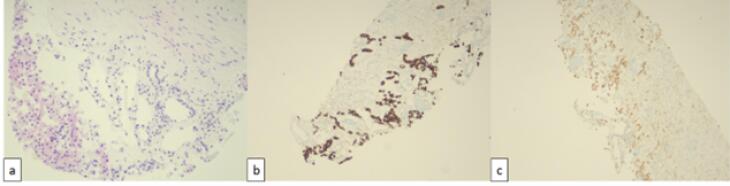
Based on these findings, we diagnosed stage IV disease ChRCC. The patient was referred for ophthalmology consultation because of ocular symptoms. A fundoscopic examination revealed limited lateral gaze in the right eye as a result of invasion of the rectus lateralis muscle in the retro-orbital region. Sunitinib was administered orally at a dose of 50 mg daily over 6-week cycles consisting of a 4-week treatment period followed by a 2-week rest period. In addition, the retro-orbital mass was targeted with palliative radiotherapy, i.e. external beam radiation therapy (EBRT) at a dose of 30 Gy. After radiation therapy, administration of systemic corticosteroids was initiated. This dramatically improved the proptosis, double vision, and ocular movements; all three symptoms disappeared within 3 months of completion of radiation therapy. MRI showed that the metastatic lesion in the right retro-orbital area had shrunk significantly (Figure 2b). However, control thoracoabdominal CT showed no significant difference in the renal mass or other metastatic foci. Given these results, the treatment protocol was changed to everolimus at 10 mg/d. The patient’s condition worsened, and he died 1 month later.
